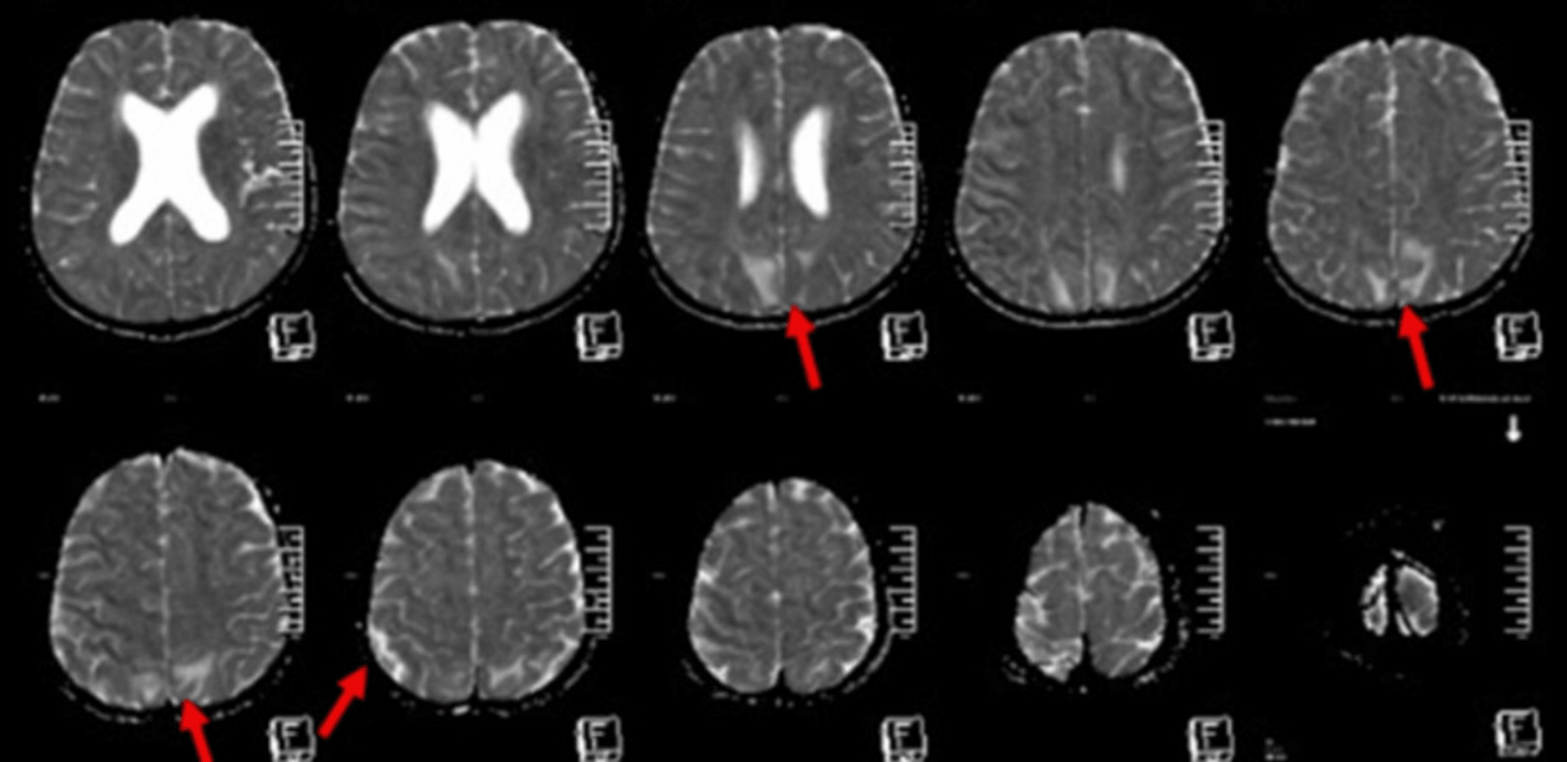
Brain MRI. Axial section showing diffuse vasogenic edema noted in the subcortical and deep white matter of bilateral parieto-occipital lobes (red arrow). MRI: Magnetic resonance imaging
